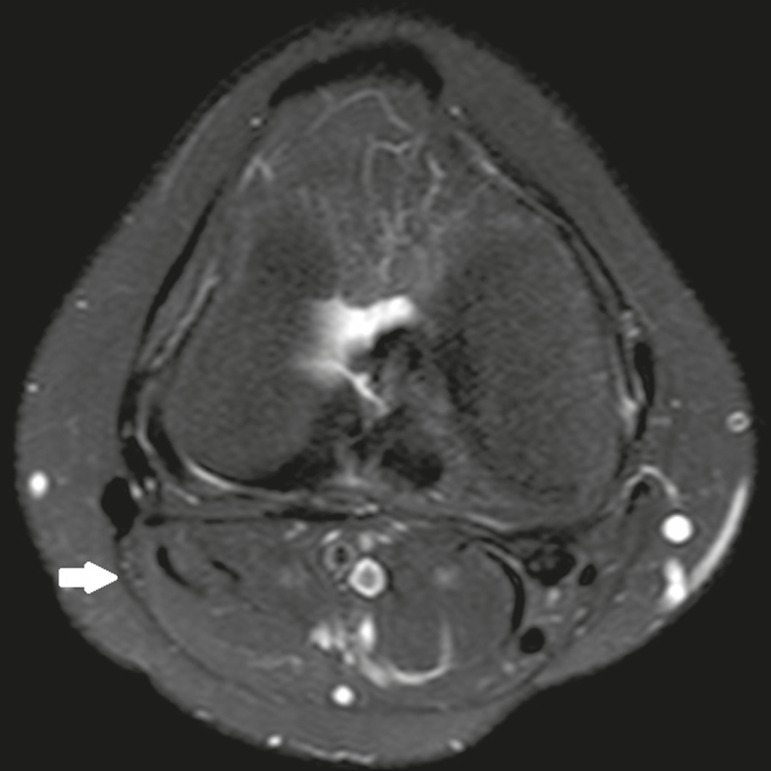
Axial T2-weighted MRI sequence with fat saturation, showing a normal CPN. The nerve presents normal signal intensity and a preserved fascicular pattern (arrow).
